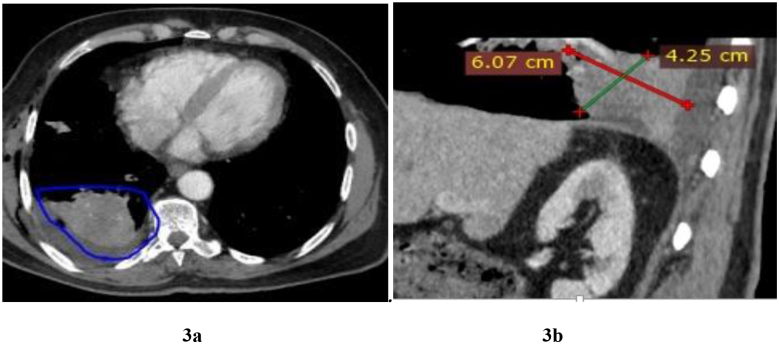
Chest CT scan; Axial and sagittal reformatted sections of chest at the level of the consolidation. Right lower lobe posterior basal segment consolidation (6.07 × 4.25) cm with no air broncograms associated with mild right pleural effusion.
